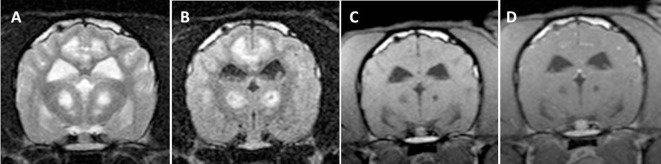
Transverse magnetic resonance images (0.5 T) of a Yorkshire Terrier (3 years, male) with subacute necrotizing encephalopathy. Bilateral symmetric hyperintensities in the cingulate gyrus and in the thalamus on T2-weighted (A) and fluid-attenuated inversion recovery (B) images. These lesions have a hypointense center on T1-weighted (C) images and they do not enhance after a contrast injection (D).
